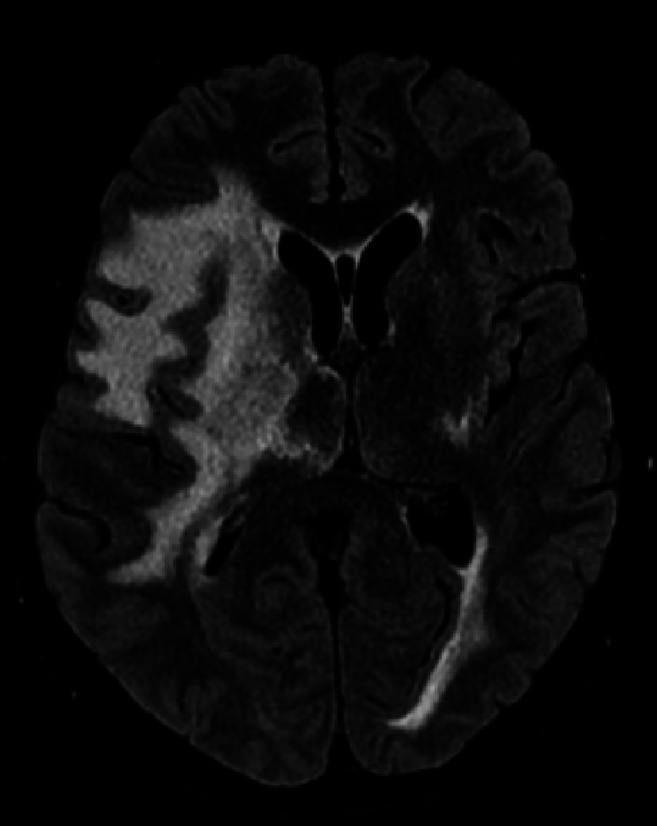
MRI brain without contrast in T2 FLAIR sequence on day 5 of hospitalization after completion of 5 dose methylprednisolone course. Imaging was remarkable for improvement of midline shift to just 3 millimeters.
